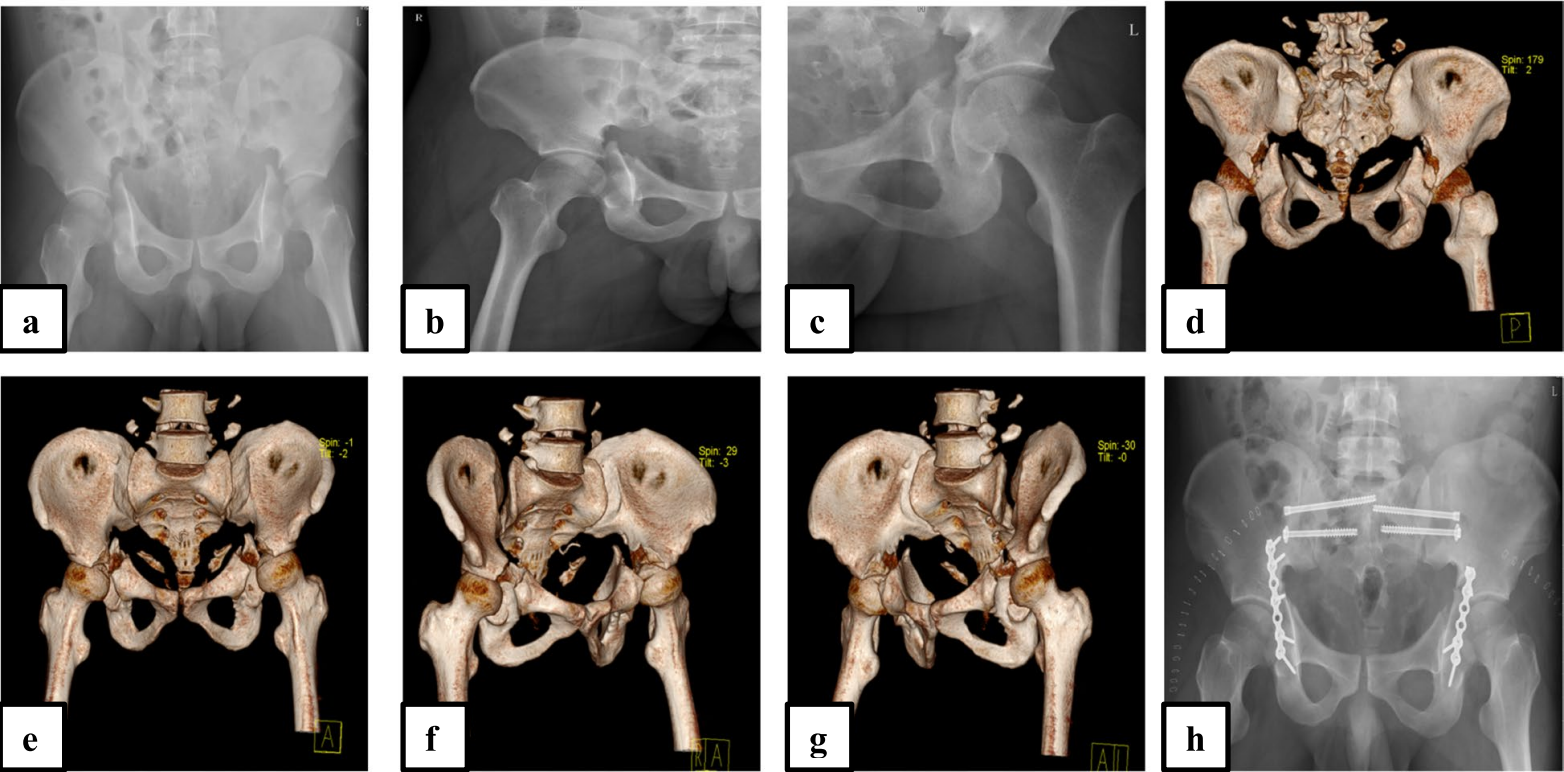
A 53 years-old male patient who sustained a bilateral acetabular fracture (Letournel-Judet transverse type) following route traffic accident: (a) preoperative plain radiograph anteroposterior view; (b) and (c) preoperative radiograph showing left and right acetabulum fracture; (d) 3D Ct scan image posterior view showing posterior displacement; (e) 3D Ct scan image anterior view showing evidence of both acetabula fracture, preoperative 3D Ct scan image showing Tile C type Pelvic fracture with bilateral sacroiliac joint dislocation (f) and (g), sacral fracture); (h) postoperative plain radiograph anteroposterior view
